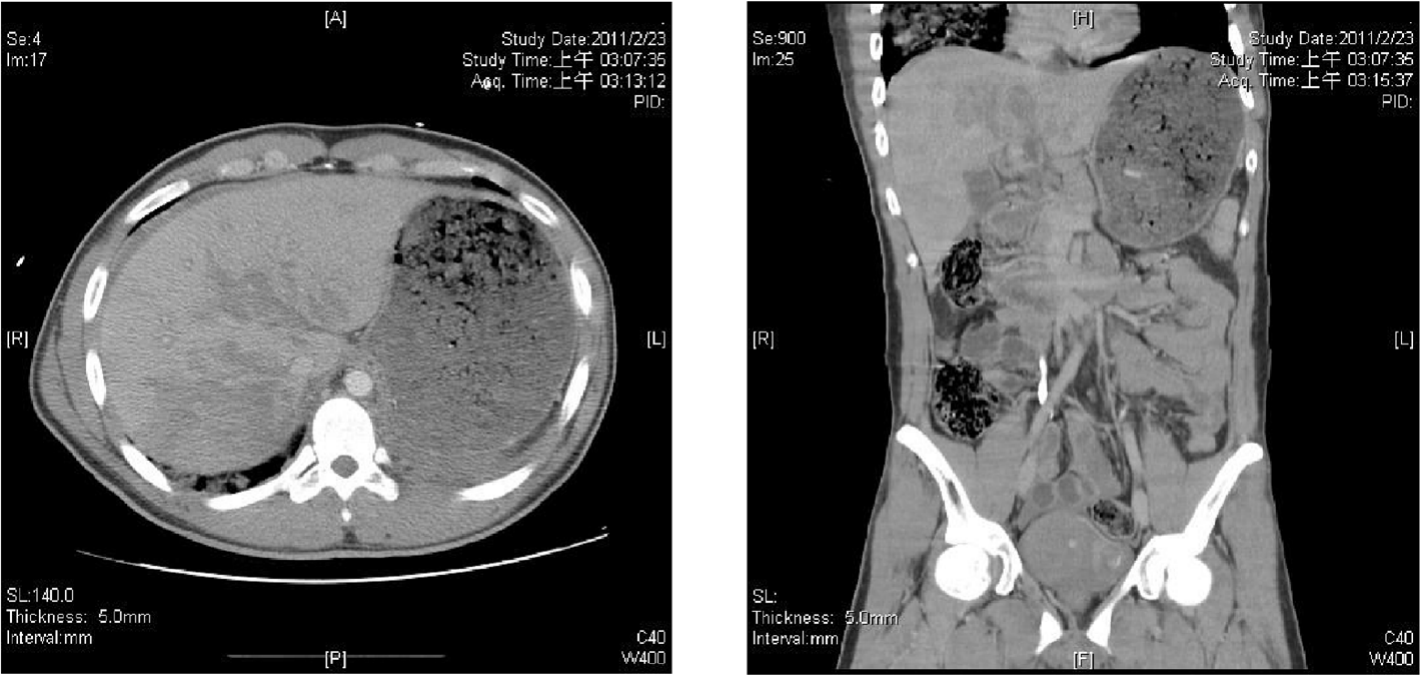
The coronal view and saggital view of abdominal CT showed grade IV laceration over bilateral hemiliver without evident contrast extravasation.
